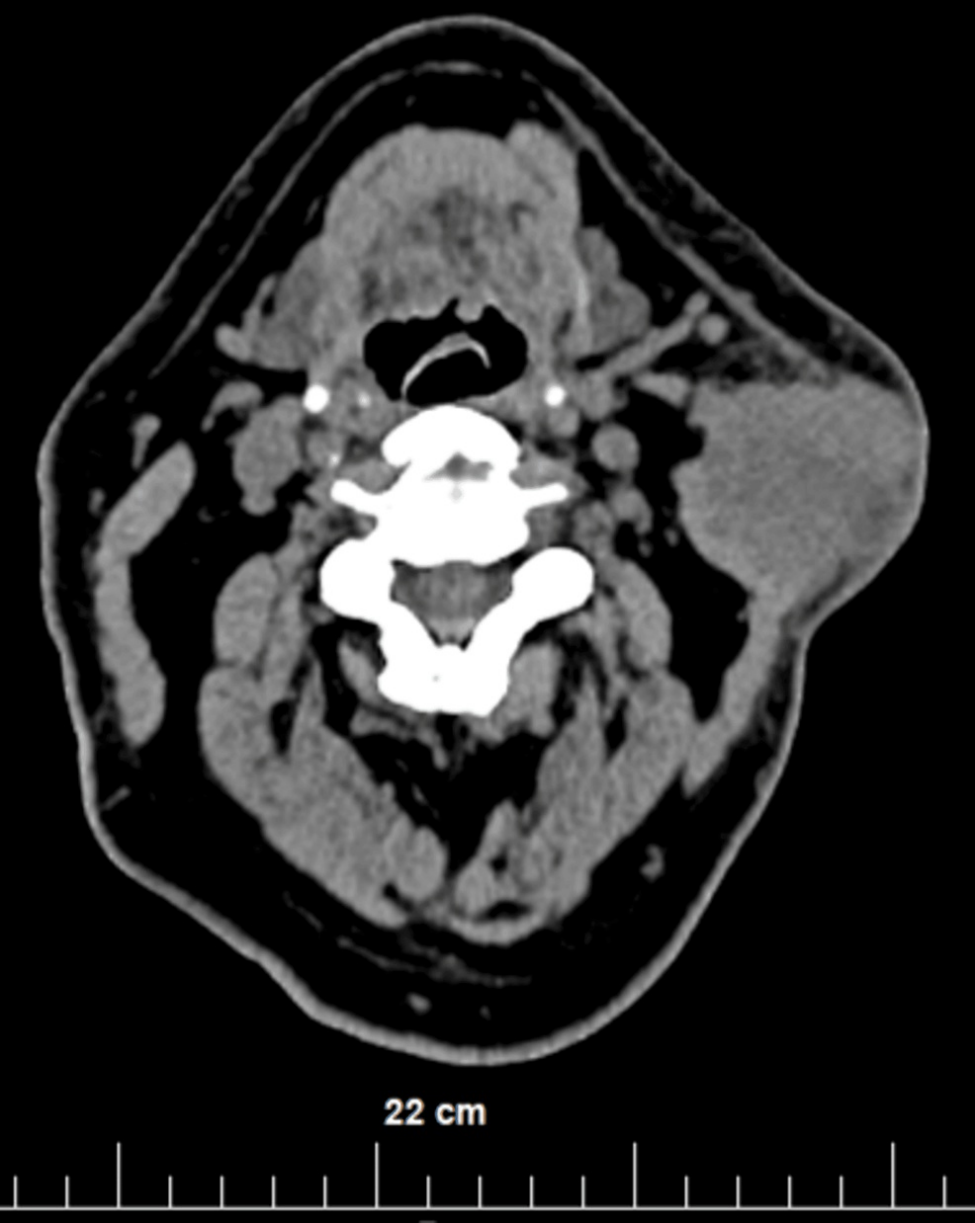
Coronal CT scan of the head and neck showing the tumor mass in the left parotid gland. The tumor mass involves the two lobes of the left parotid gland and the facial nerve between them. The Ill-defined margins demonstrated by the lesion are strongly suggestive for malignancy.
